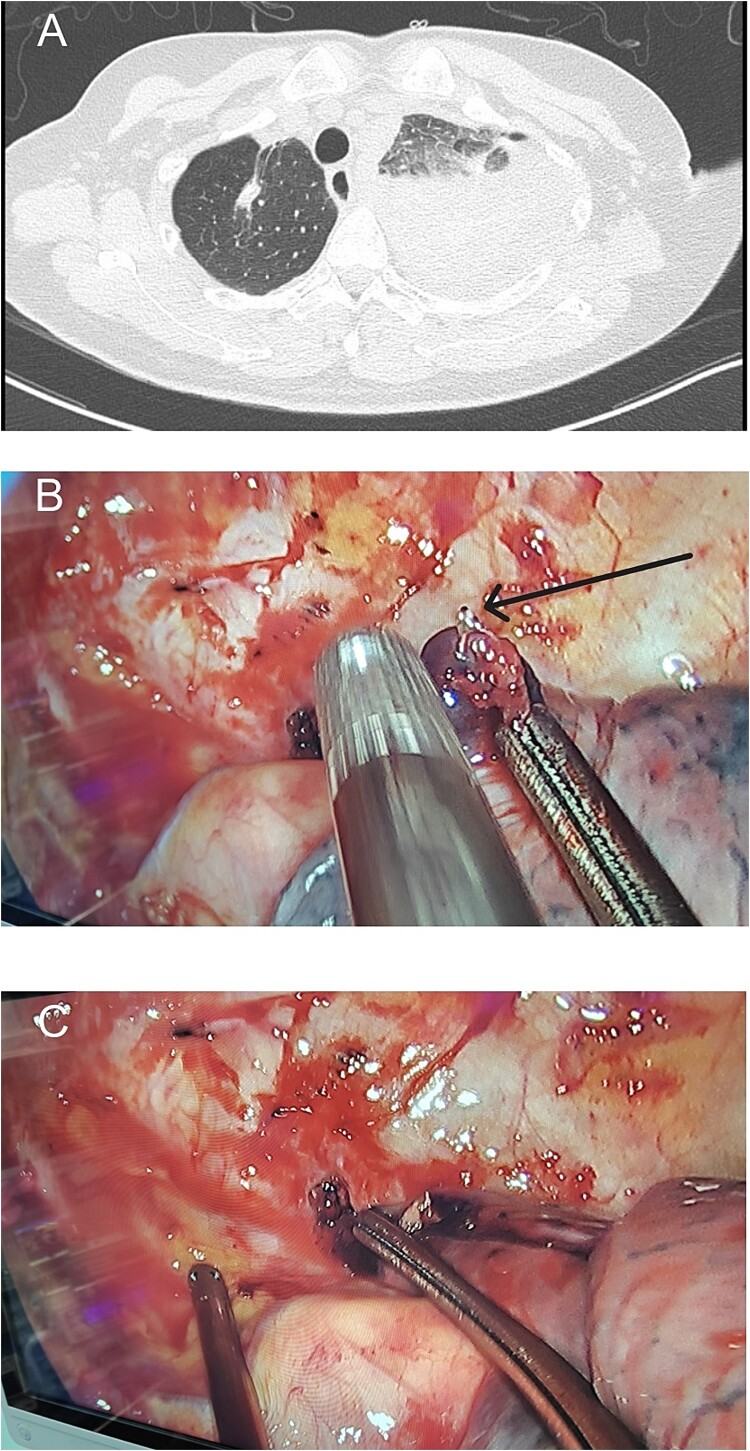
Preoperative chest computed tomography and intraoperative findings on postoperative day 3. (A) Computed tomography shows a large amount of fluid in the left pleural cavity, indicative of postoperative hemorrhage. (B) A protruding staple (black arrow) is observed at the resection margin of the left upper lung, near the site of chest wall bleeding. (C) During surgery, we attempted to reproduce the position that caused the bleeding.
